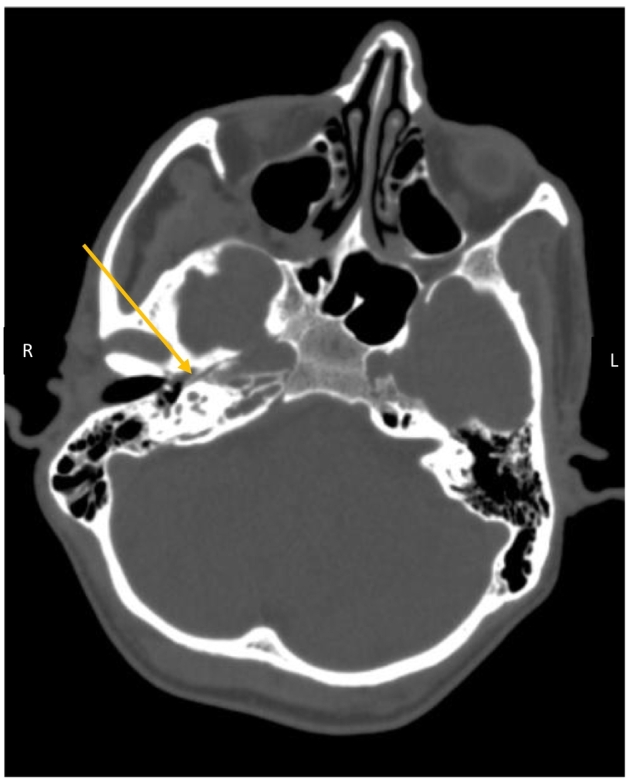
CT Brain (plain) shows suspicion of right sided petrous apicitis and otitis media.
